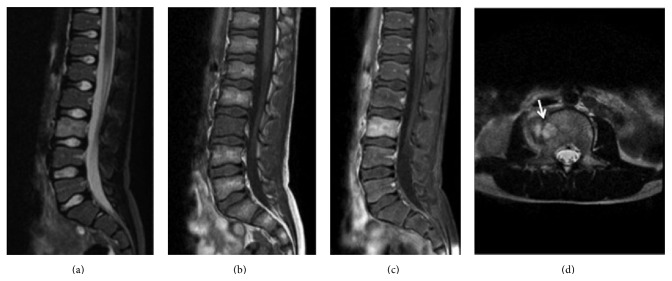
MRI scan of the lumbar spine. Sagittal spin-echo T2 weighted MR image (a) and T1 weighted MR image (b); contrast-enhanced T1 weighted image with fat saturation (c) only reveal edematous changes of the vertebral body of L3 with integrity of the L3-L4 disk. Axial Short tau inversion recovery MR image (d) shows vertebral abscess with extension to the paravertebral soft tissues (white arrow).
